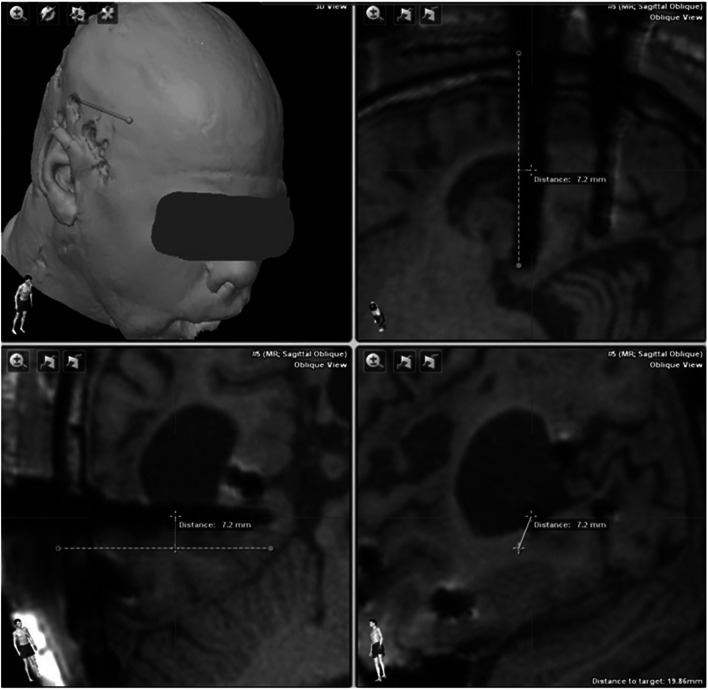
Exemplary accuracy measurement of centre point deviation. The Euclidean distance from the planned preoperative trajectory to the placed electrode in postoperative MRI is demonstrated for the centre point (CP) of a single electrode
